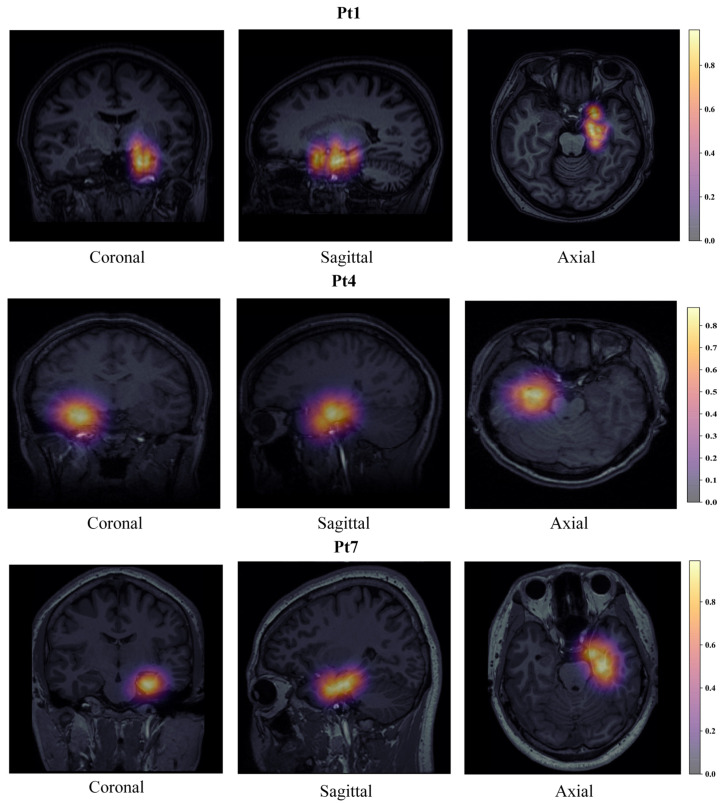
The SOZ prediction and visualization result with MRI from multiple axes.
